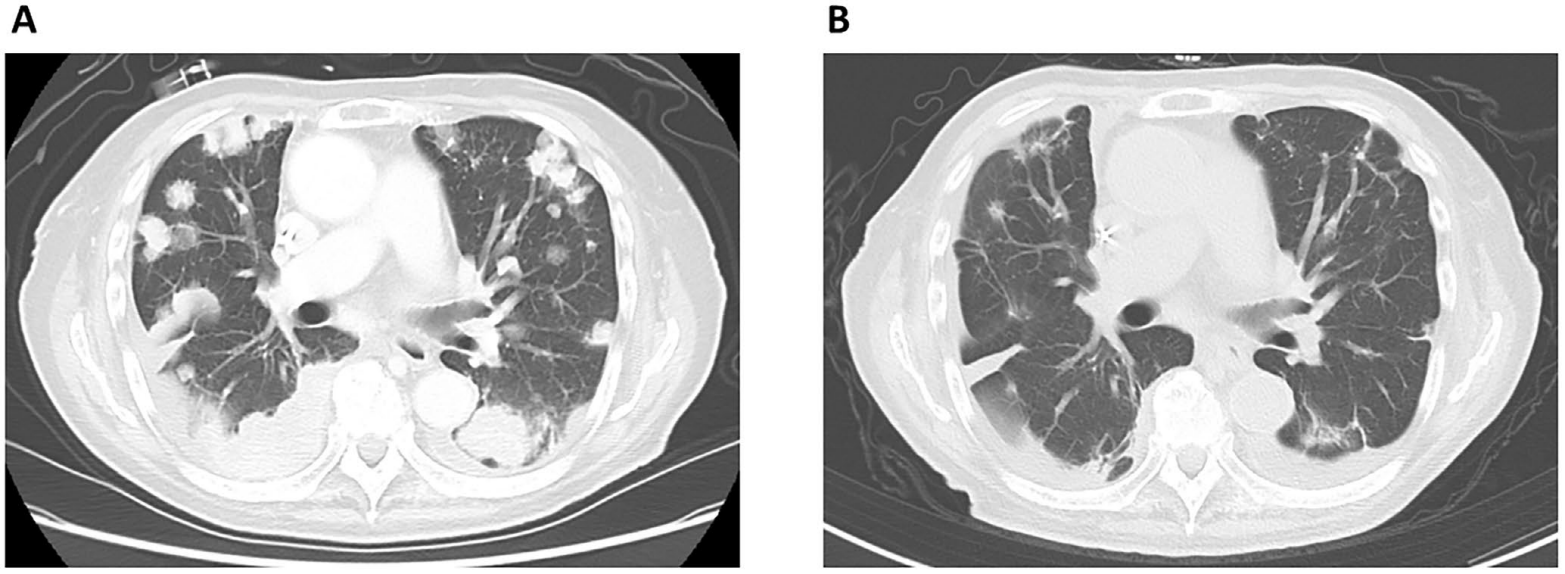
(A) After four cycles of avelumab maintenance therapy, plain chest CT shows the multiple lung tumors growth, which are more advanced than before first‐line chemotherapy. (B) After two cycles of EV therapy, plain chest CT shows significant shrinkage of the multiple lung tumors.
